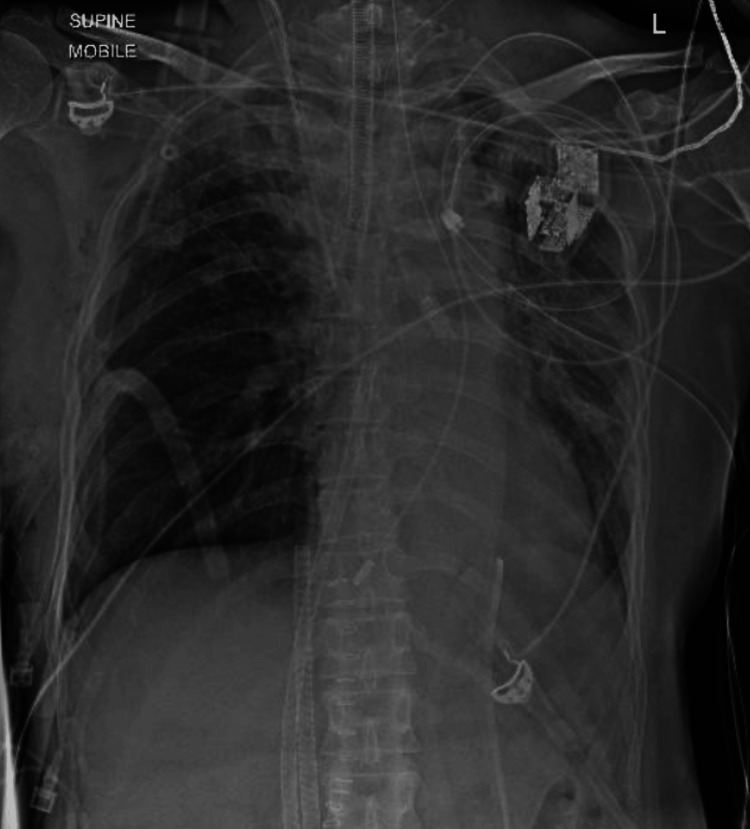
Post-operative chest X-ray showing the final position of the armored ETT. ETT, Endotracheal Tube
